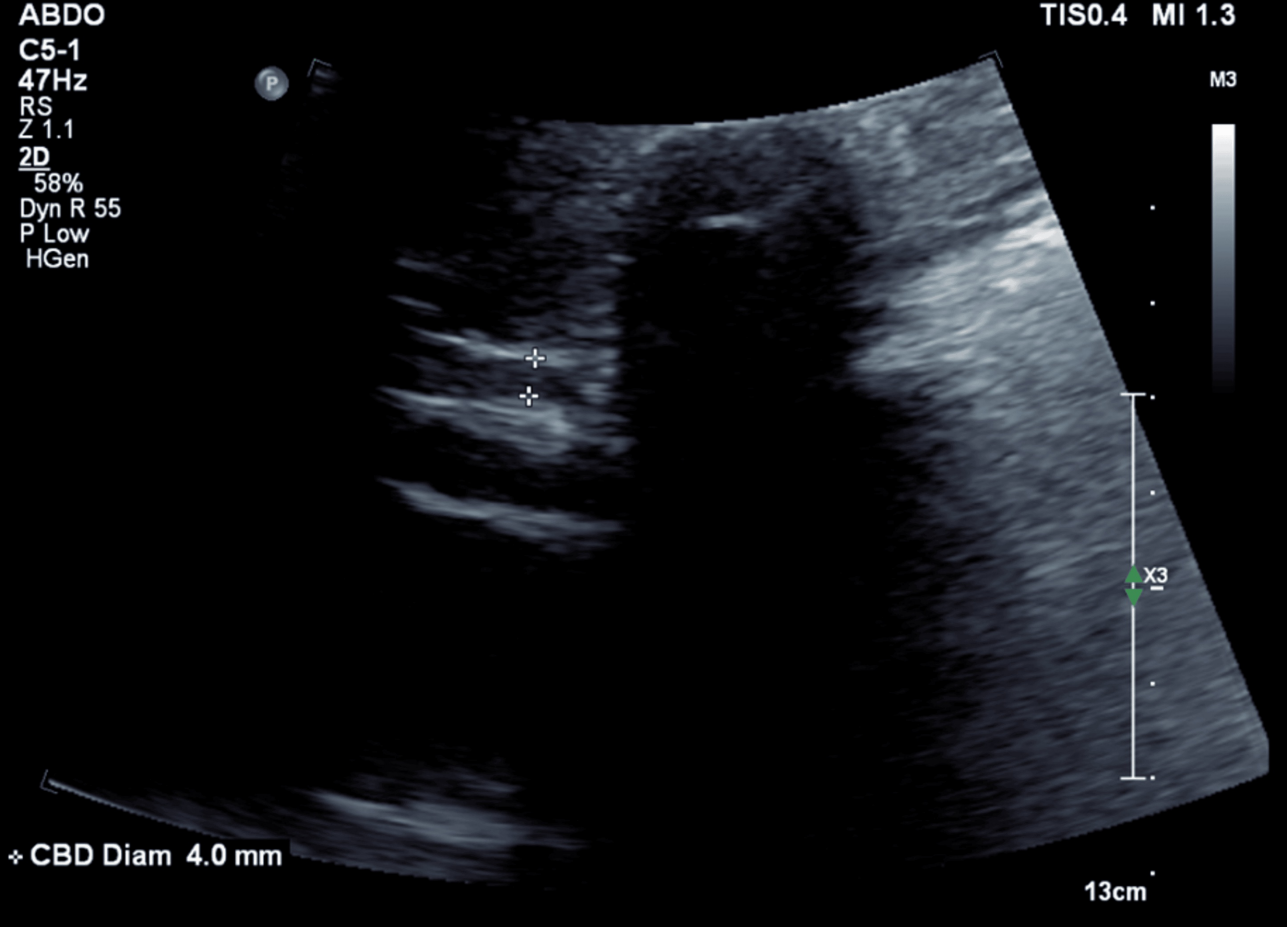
Ultrasound demonstrates a common bile duct diameter of 4.0 mm, consistent with a non-dilated biliary system.
